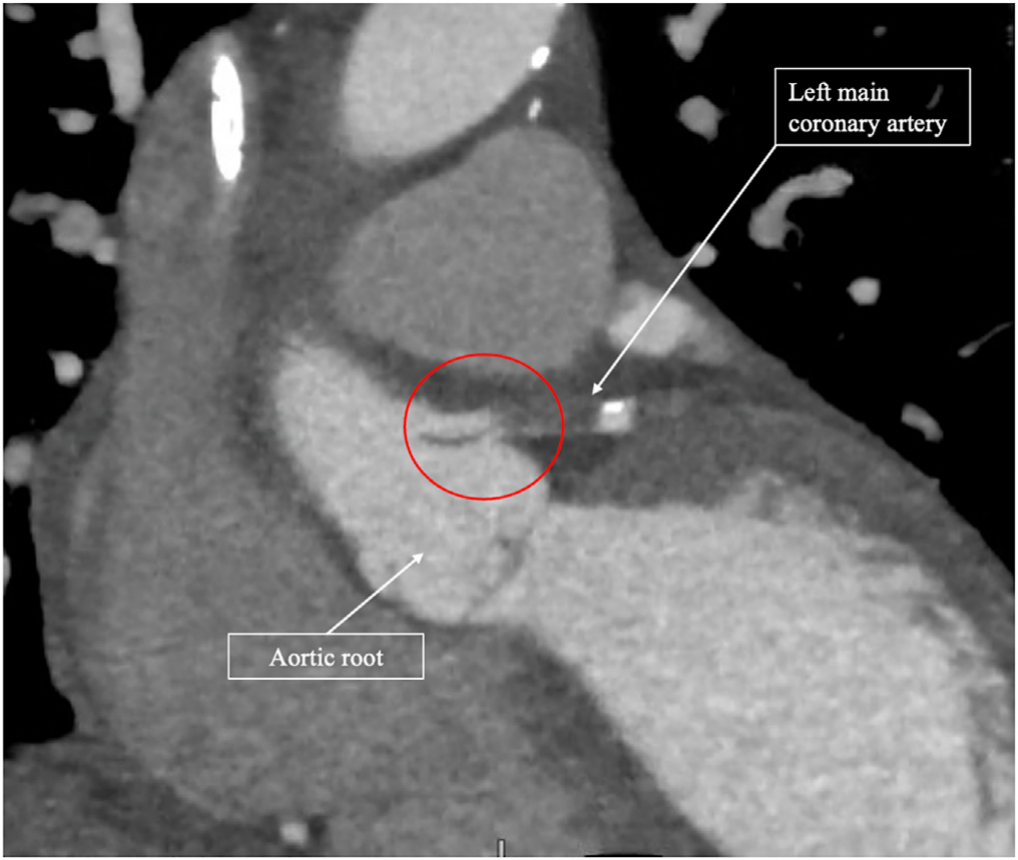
CTA gated thoracic aorta revealing a type A aortic root dissection (red circle) extending into the left main coronary artery with subtotal occlusion. CTA, computed tomography angiography.
